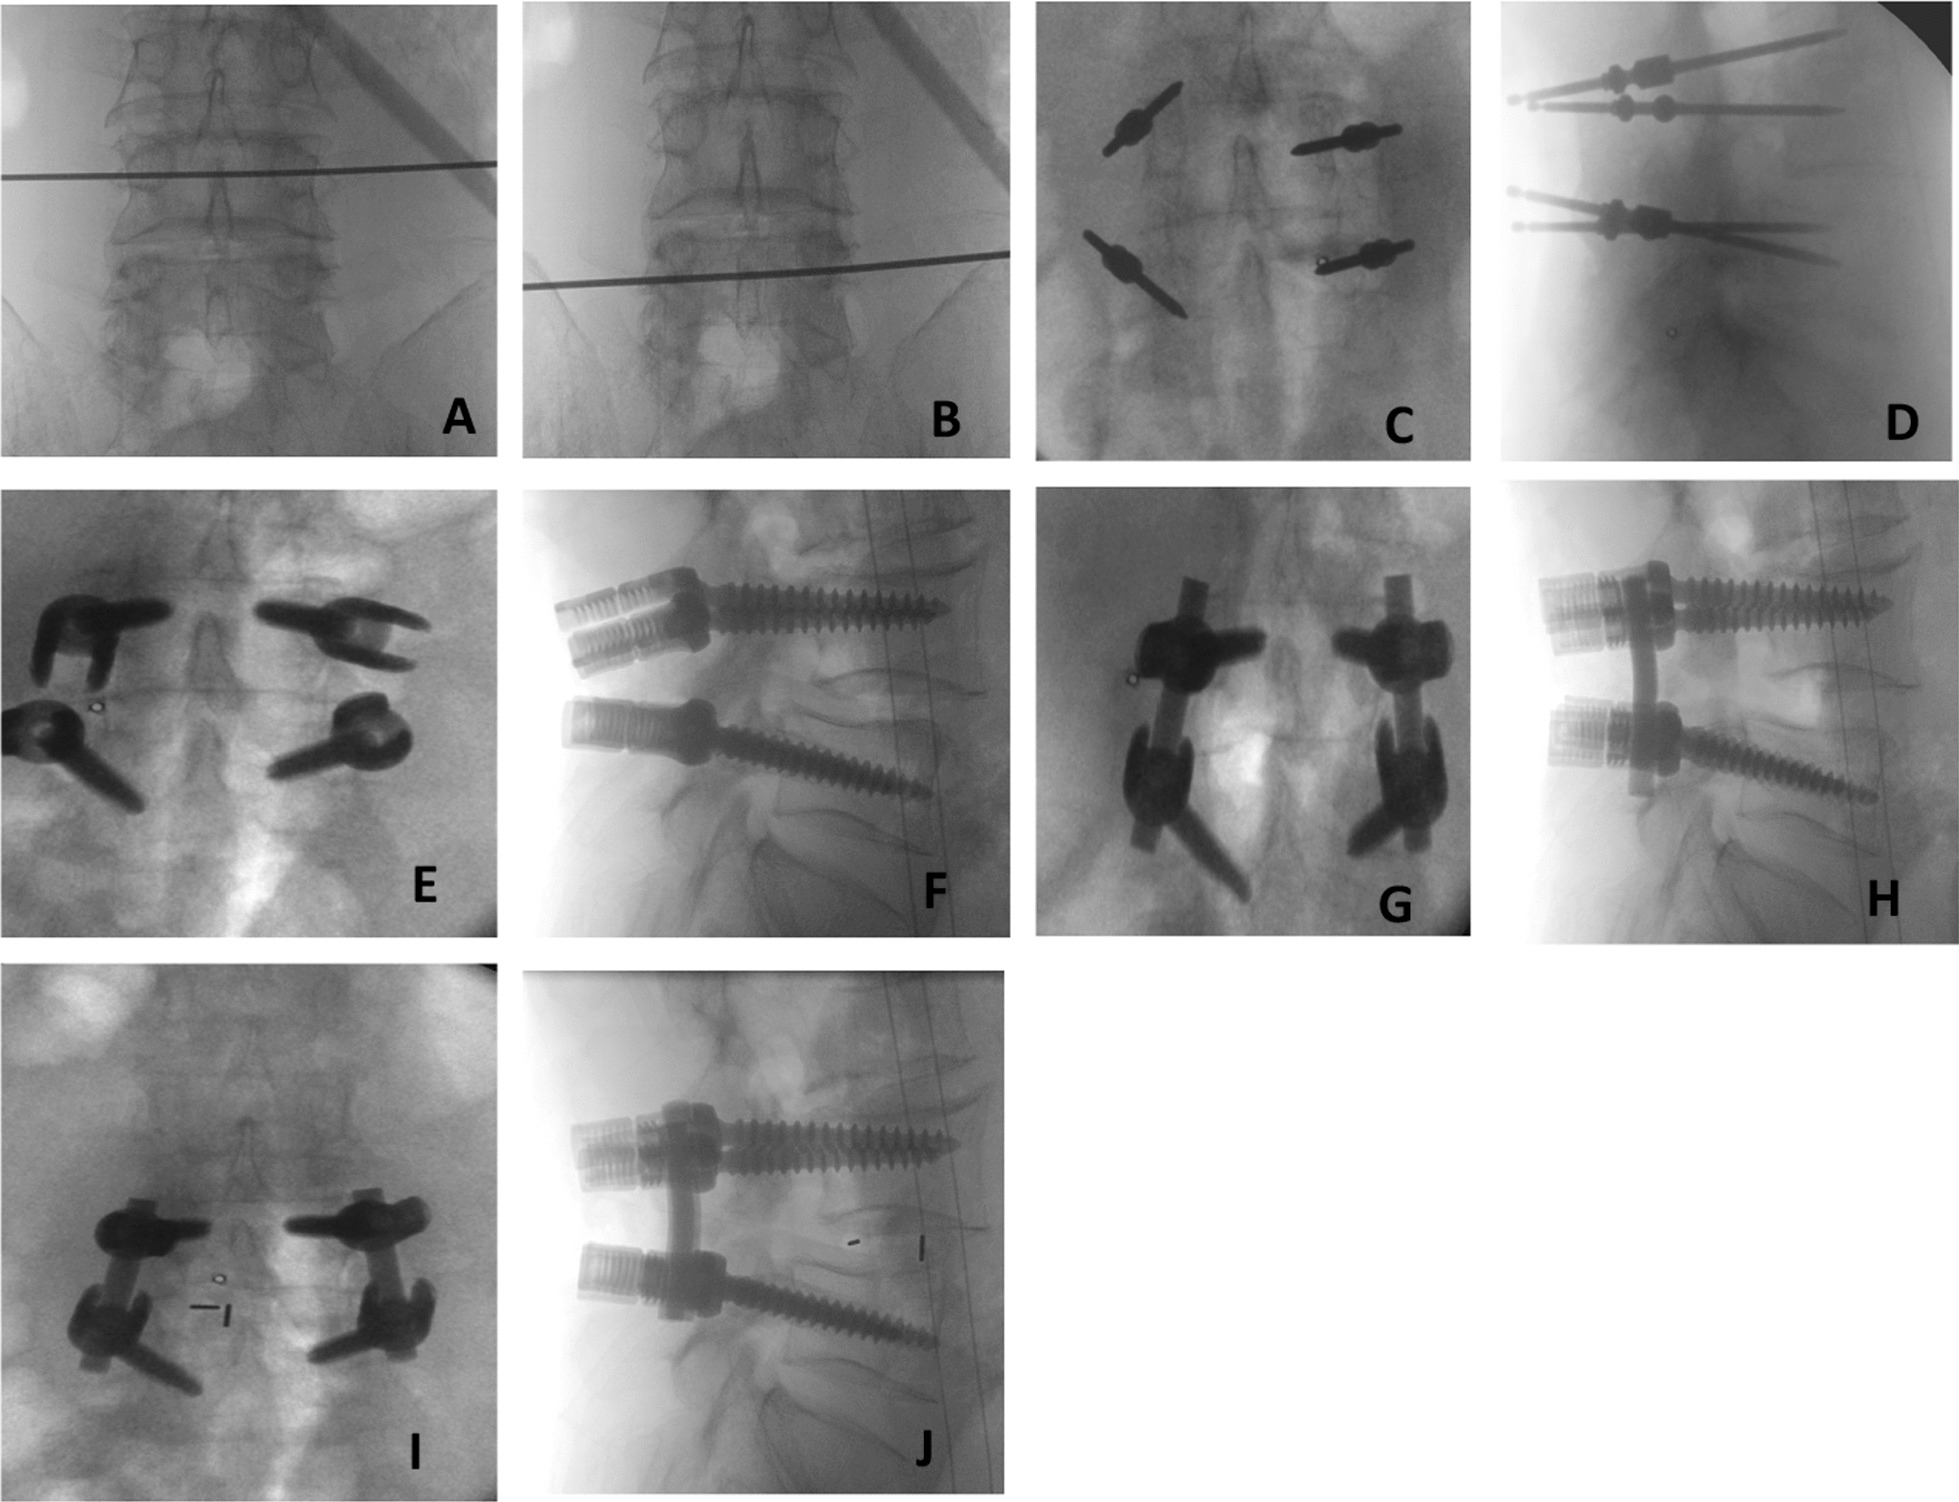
The procedure of M-TLIF operation. L4 (A) and L5 (B) vertebrae were identified under X-ray machine fluoroscopy; Kirschner wires were implanted along the pedicles of L4 and L5 vertebrae (C, D); After adjustment, pedicle screws of suitable size (E, F) were placed; suitable connecting rods were cut and fixed, laminae were opened, articular processes were cut off, and intervertebral discs were decompressed (G, H). A suitable interbody fusion cage (I, J) was filled from the degenerative side to restore the interbody height and the position of the cage was satisfactory
